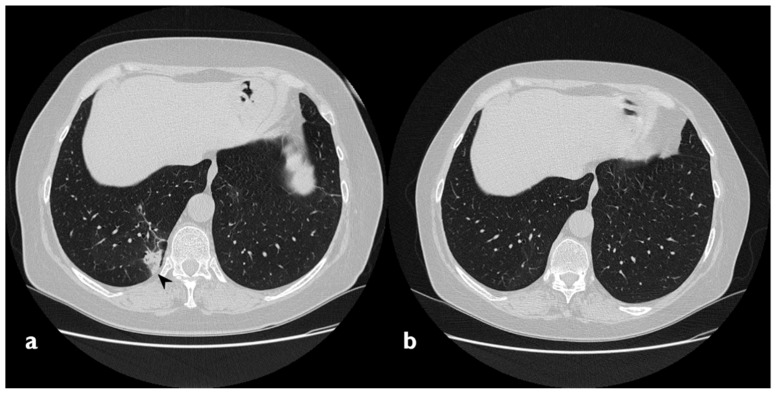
On the left: baseline HRCT (a); subpleural consolidation in the right lower lobe. On the right (b): HRCT after 11 months of steroid therapy shows a disappearance of the consolidation.
